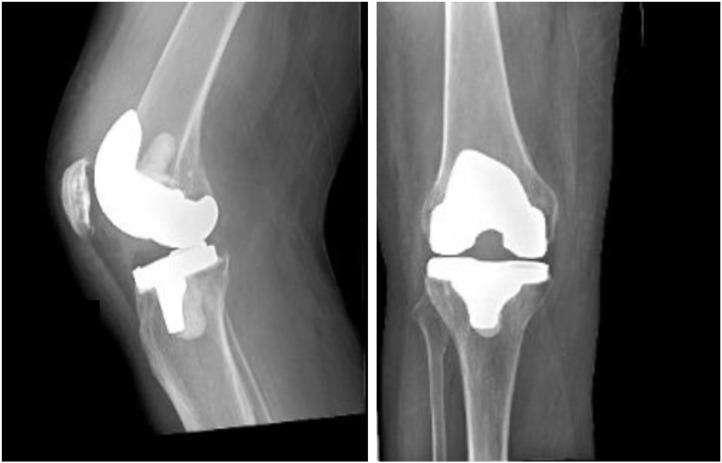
The preoperative radiograph showed the right total knee replacement with the prosthetic components.
